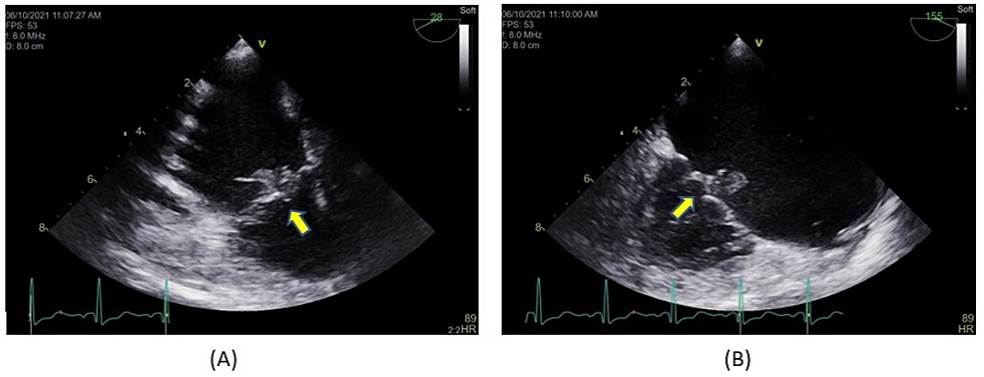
Parasternal long-axis view on transesophageal echocardiogram shows vegetation measuring 7.8 mm x 5.6 mm on the atrial aspect of the septal leaflet of the tricuspid valve (arrows)
